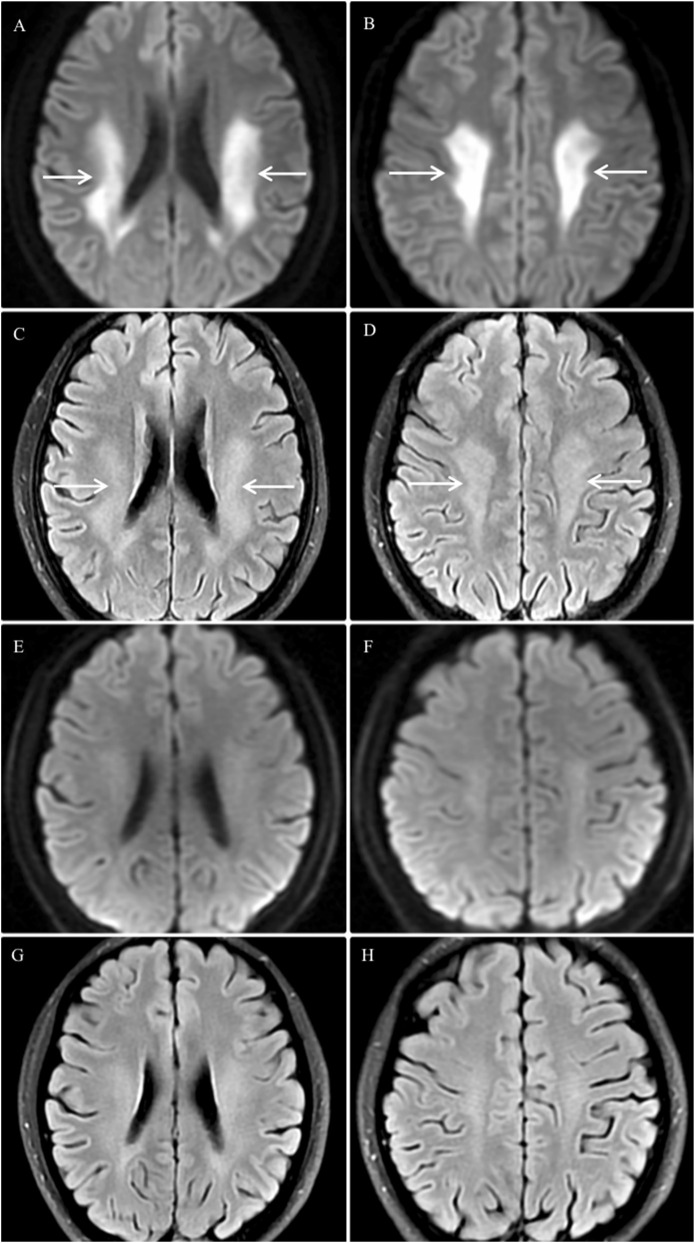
Brain MRI findings. (A–D) Acute Phase: Brain MRI on the third day after the final episode demonstrated bilateral symmetric restricted diffusion (arrows) in the periventricular deep white matter and centrum semiovale on DWI (A,B), with corresponding hyperintensity (arrows) on FLAIR images (C,D). (E–H) Resolution Phase: DWI (E,F) and FLAIR (G,H) sequences obtained 1 month later showed near-complete resolution of the aforementioned abnormalities.
